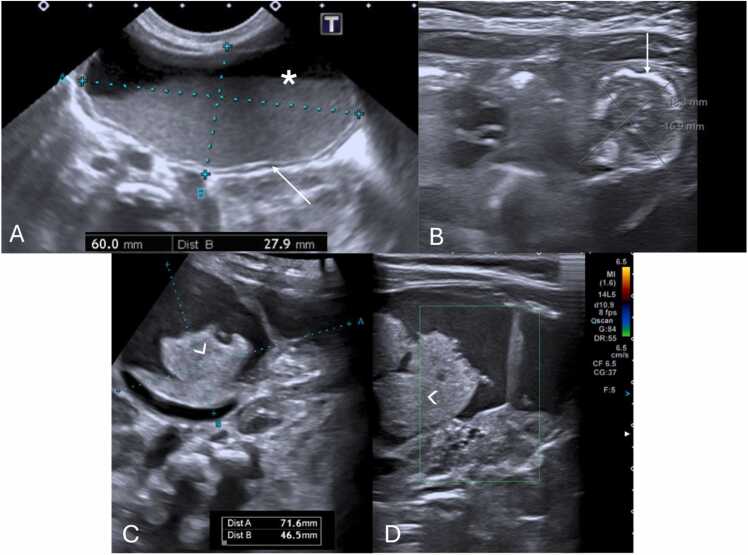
Neonatal ovarian torsion in a 3-day-old infant with a prenatally diagnosed cystic mass with a fluid-debris level. Transverse transabdominal pelvic US image (A) obtained at the 3rd day of life shows a cystic mass (between calipers) with fluid-debris levels (* in A), in the left pelvic region. It was surgically confirmed to be a left ovarian torsion with cystic fluid separated from liquefied hematoma (no pathology images available). Note the presence of an echogenic inner wall and hypoechoic outer wall (arrow in A), typical for the double wall sign, suggesting infarction. (B) Transverse transabdominal pelvic US image of another surgically proven ovarian torsion in a 2-month-old infant who presented with fussiness, shows a rounded soft-tissue mass (between calipers in B) with echogenic peripheral calcifications (arrow in B) seen, suggesting infarction. (C and D) Transabdominal sagittal and transverse pelvic Ultrasound with color Doppler in a 2-week-old infant with a palpable mass in the lower abdomen region, showing a complex cystic (between calipers in C) with thick septa (arrowhead in C and D) without vascular flow and with retractable cloth sign. This mass was described in an obstetric ultrasound as a simple cyst (no previous images available). During exploratory laparoscopy it was confirmed to be a left ovarian torsion.
